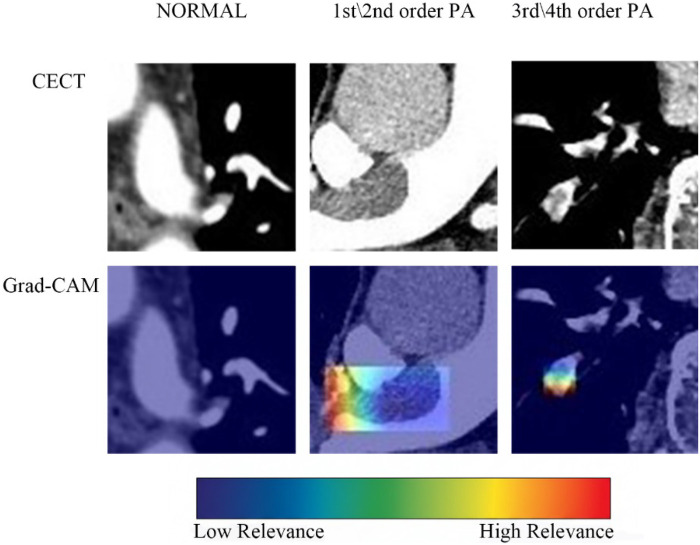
Visualization results of Grad-CAM across different grades. CECT, contrast-enhanced CT; PA, pulmonary artery; Grad-CAM, gradient-weighted class activation mapping.
